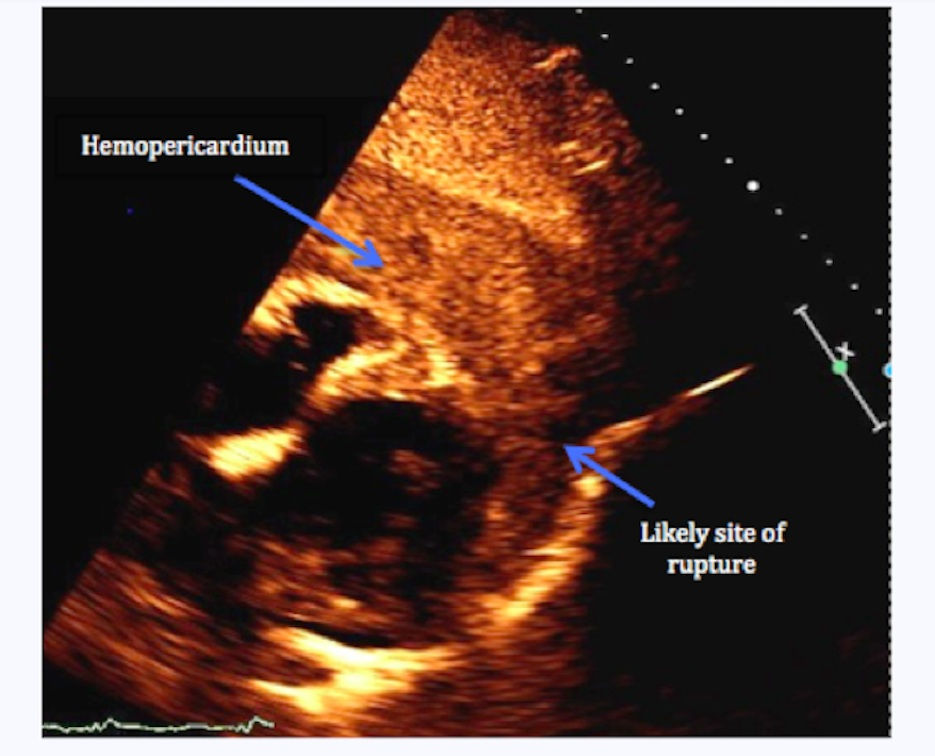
Echocardiogram showing the site of myocardial rupture and the resulting hemopericardium
